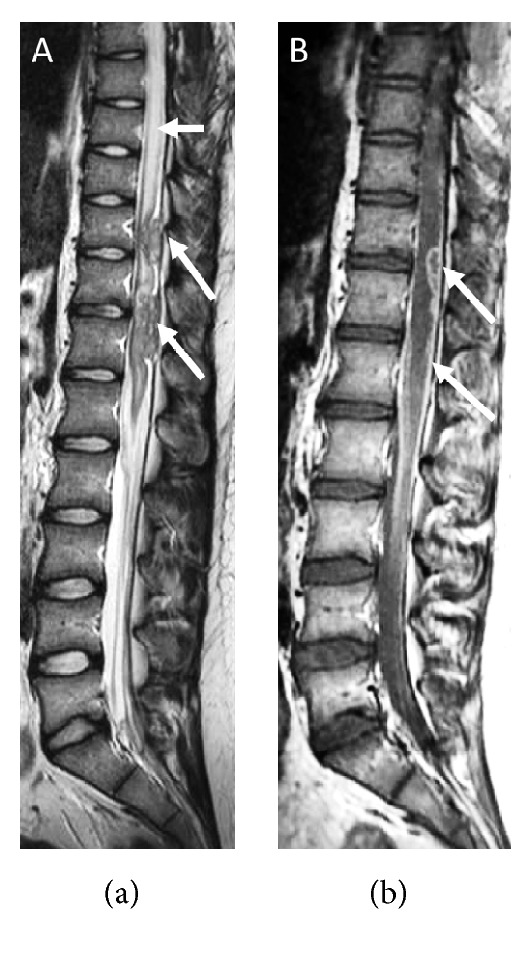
Sagittal FSE T2 (a) and postcontrast T1 (b) MR images of thoracolumbar spine at about 3 months of first admission. Spinal cord edema (Arrow 1). Diffuse nodular leptomeningeal and dural disease with ring enhancing abscesses (Arrow 2).
